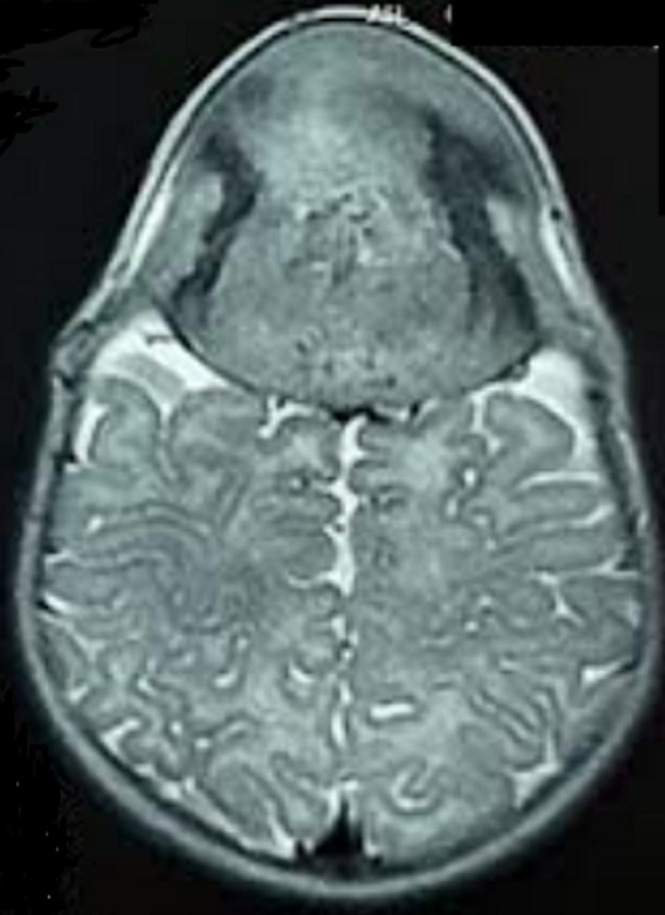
cerebral magnetic resonance imaging axial plane in T2-weighted sequence
